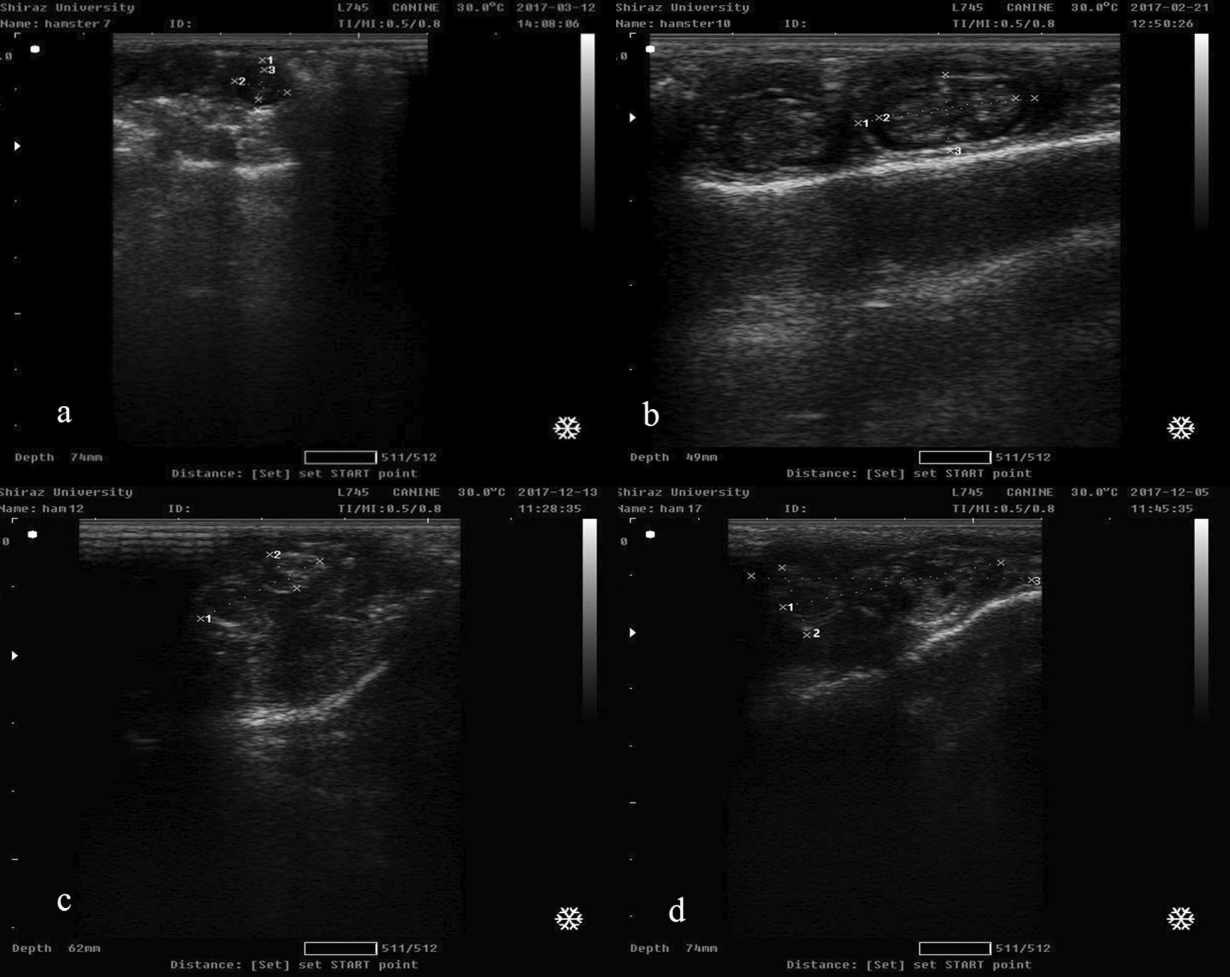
The ultrasound images on days 7 (a), 10 (b), 12 (c), and 17 (d) of pregnancy in golden hamsters. Crown-rump length (CRL), gestational sac diameter (GSD), and head diameter (HD) were used to estimate the gestational age of golden hamsters
